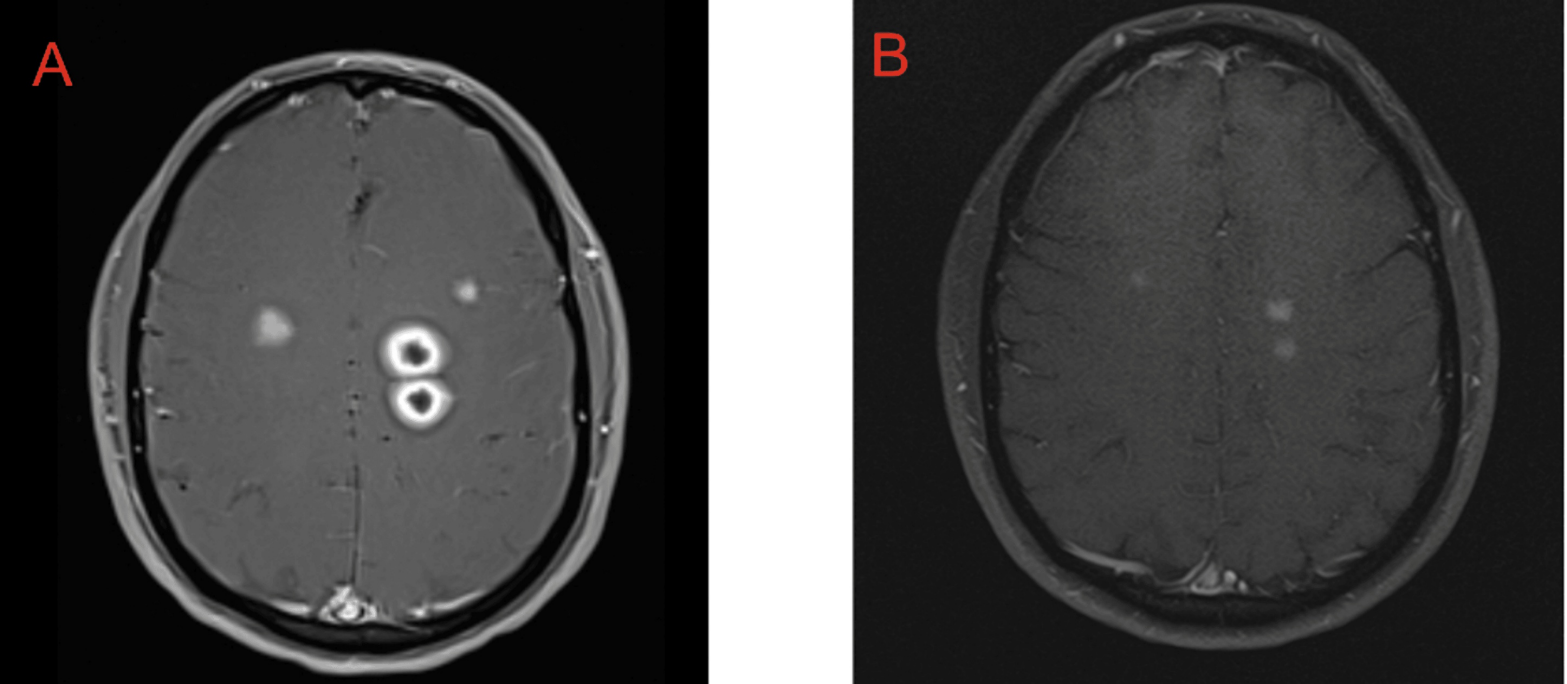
MRI brain with contrast, axial views, T1 weighted. Images from day 4 in the hospital (A) and three months later (B) show a significant reduction in lesions.
